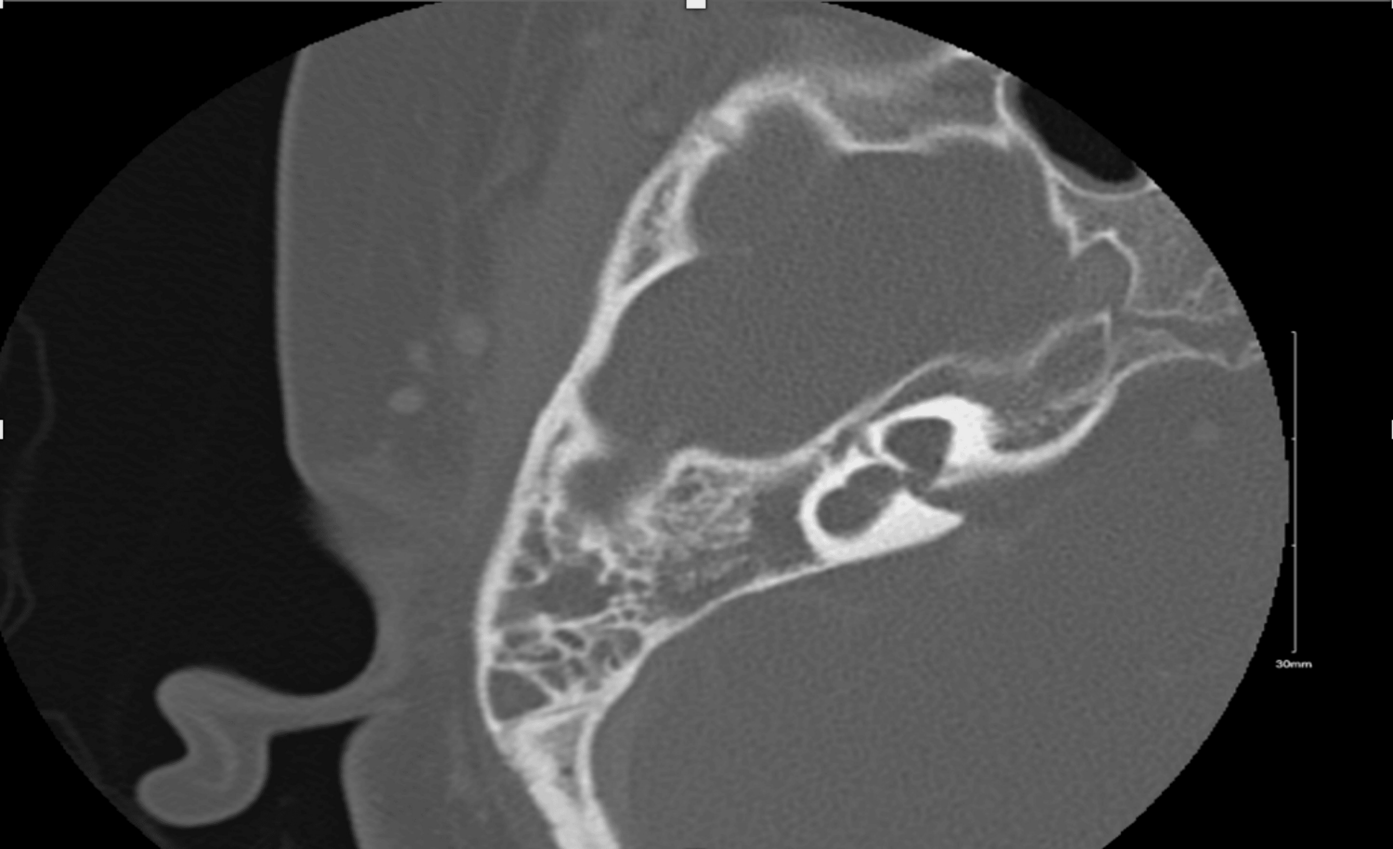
CT shows inner ear malformation with cystic dilatation of the cochlea and vestibule and absence of the semicircular canal. There is also a middle ear and mastoid effusion. The vestibular aqueduct and internal auditory canal are normal
